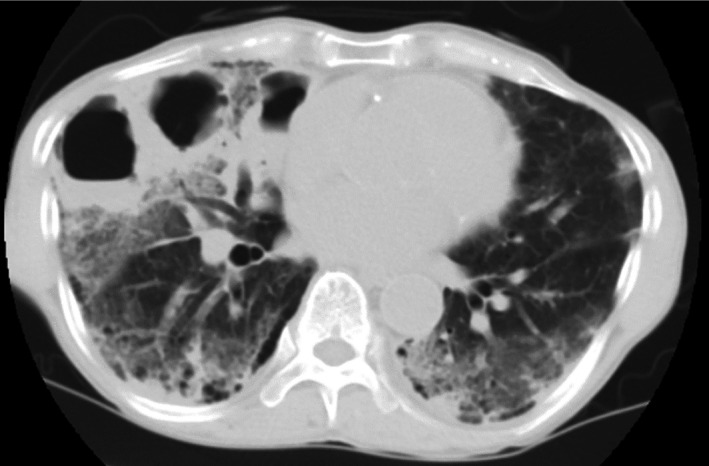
Chest computed tomography scan obtained three days after admission, showing multiple giant cavitary lesions with air‐fluid levels in the right lower lung lobe
